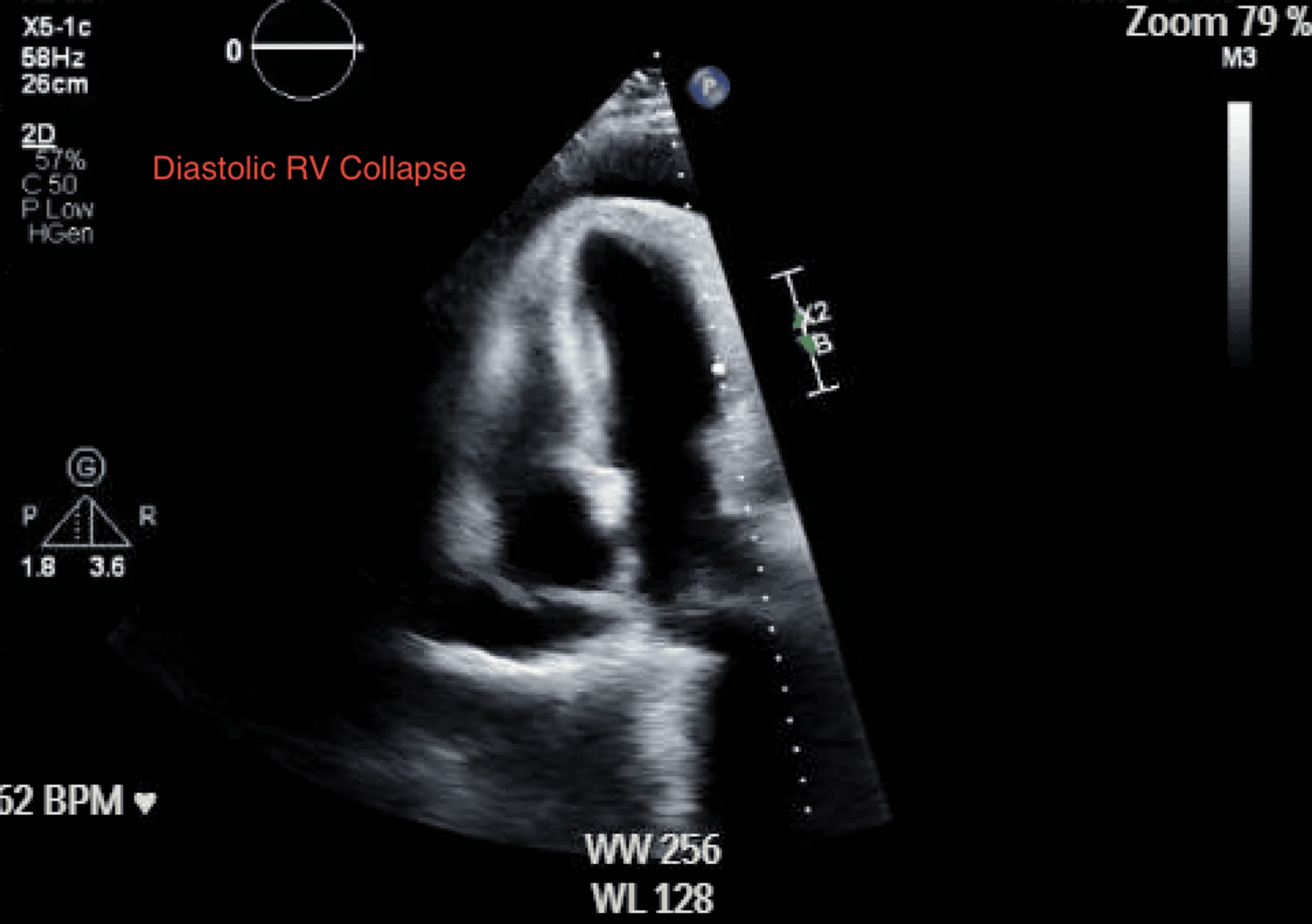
Transthoracic echocardiography showing early diastolic RV free-wall collapse consistent with tamponade physiology. RV: right ventricular
